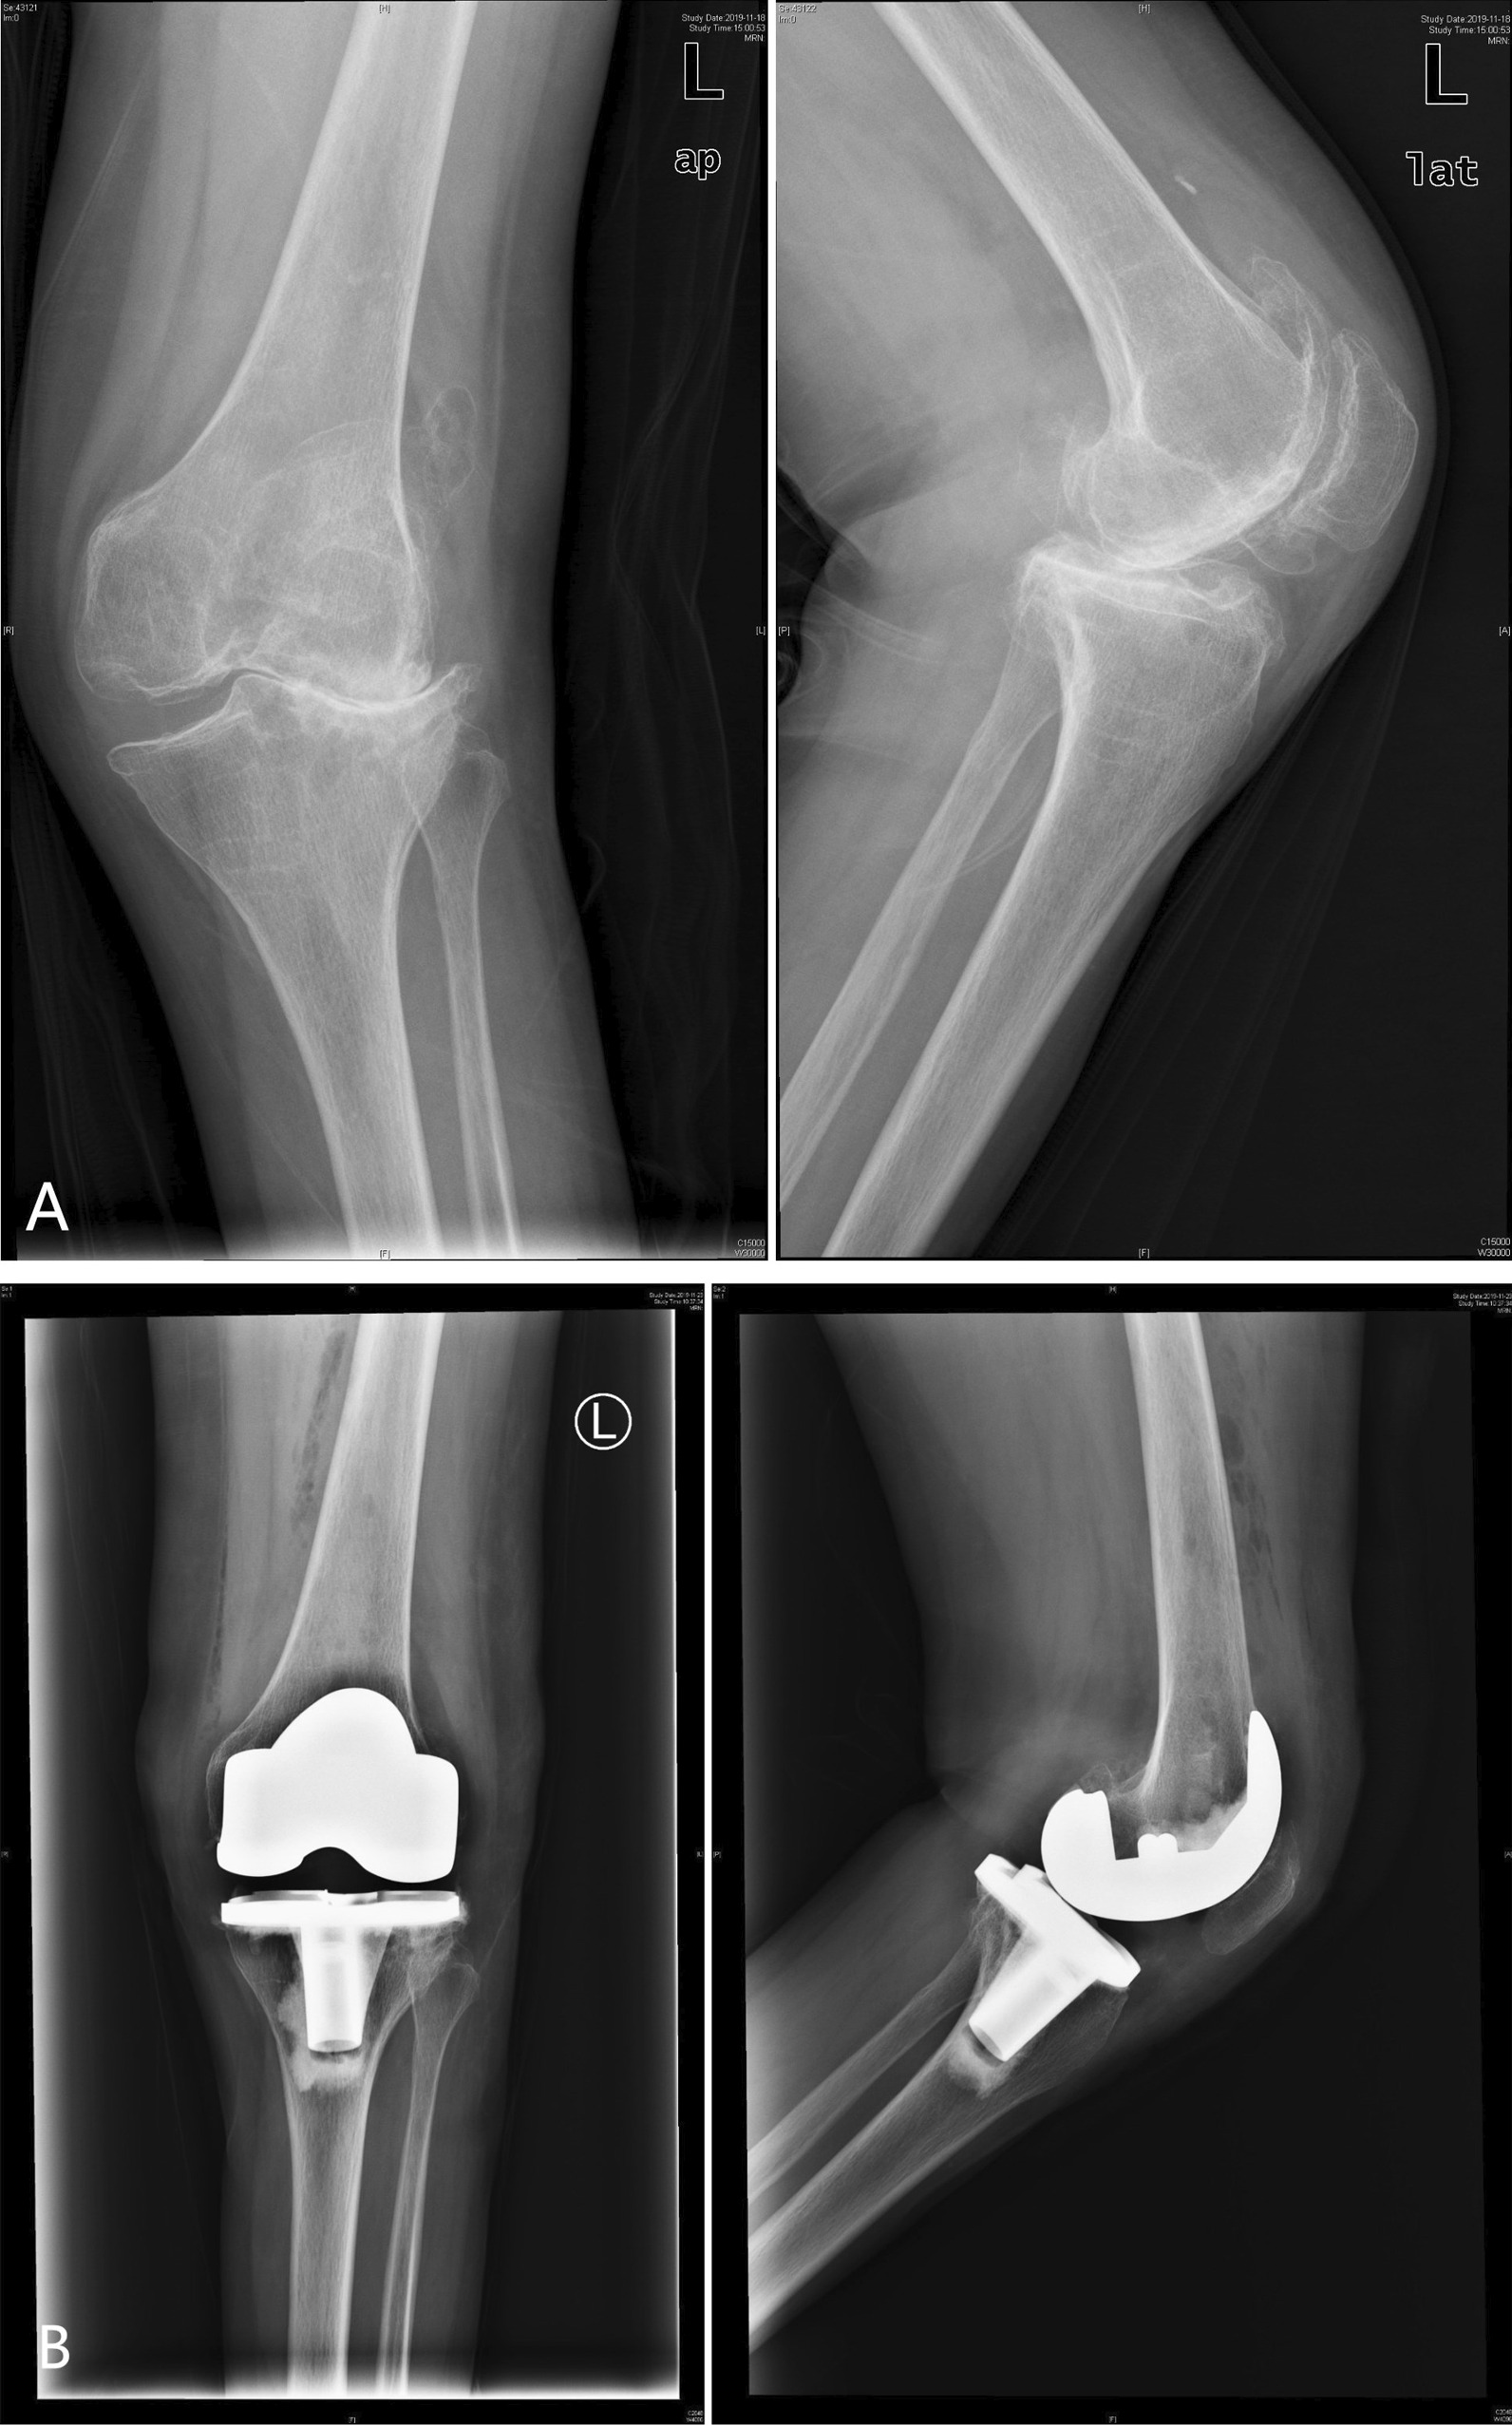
The anteroposterior and lateral knee radiographs of left knee before and after the operation. A preoperative valgus knee with osteophyte; B postoperative knee status
